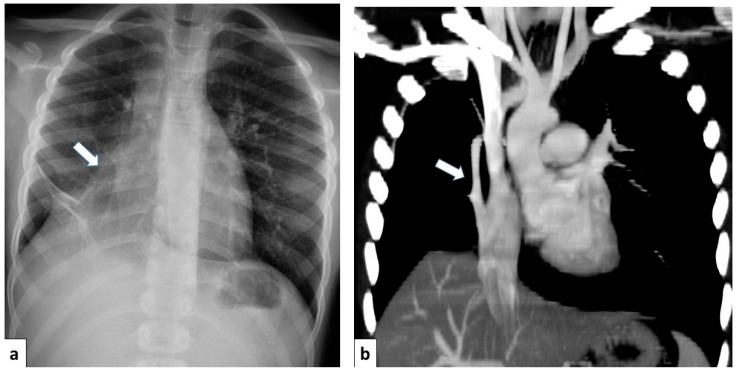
(a) Chest radiograph of a 11 year old boy shows an anomalous vein appearing as a curvilinear opacity (arrow) in the right lower lobe, similar to a scimitar sword; note that the right lung is hypoplastic; (b) CT angiography with coronal MIP reconstruction demonstrates the entirety of the anomalous scimitar vein and its associated drainage in the inferior vena cava (arrow).
